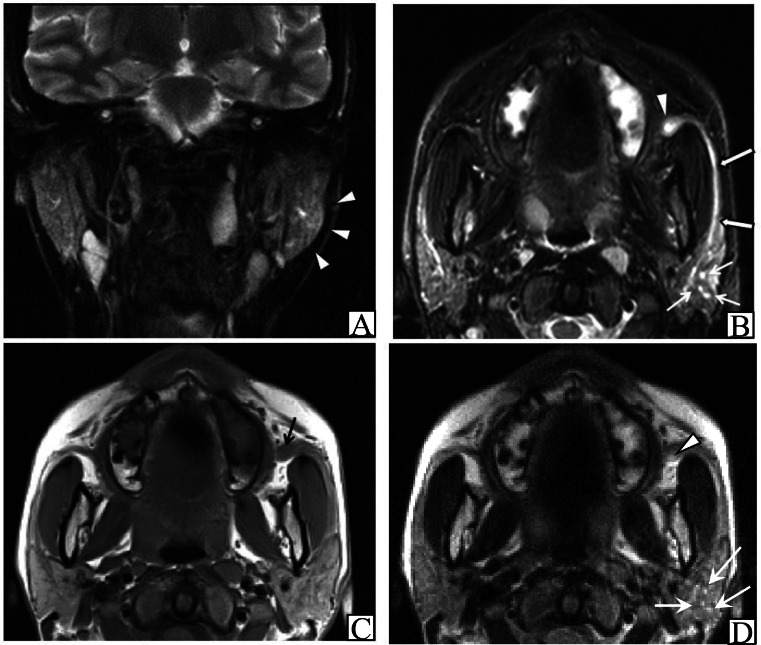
MR images. (A) Coronal STIR image shows obvious swelling of the left parotid gland. (arrowheads). (B) Axial STIR image shows multiple punctate high-signal areas in the body of the left parotid gland (short arrows). Stensen's duct is dilated, partly stenotic, and irregular (long arrows). There is cystic dilation of the orifice due to stenosis near the left Stensen's duct orifice (arrowhead). No opacity is apparent in left-sided periparotid adipose tissue. (C) Axial T1-weighted image shows dilation of the left lateral Stensen's duct orifice (arrow). (D) Axial T2-weighted image reveals multiple spots of high signal intensity within the body of the left parotid gland (arrows). There is high-signal cystic dilation near the orifice (arrowhead).
